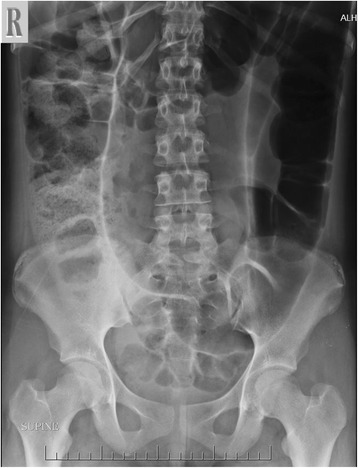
Plain radiograph of the abdomen of a 13-year-old girl showing hugely dilated loop of bowel, arising from her pelvis. It has the appearance of a coffee bean. Frimann Dahl’s sign is positive with three dense lines converging toward site of obstruction. Her rectum still contains air
